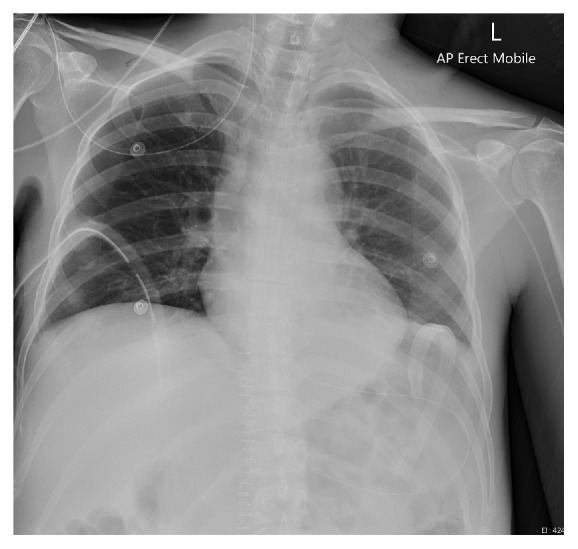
Chest X-ray showing the PICC line in the subclavian vein. Also shown is the R sided chest drain in situ.
